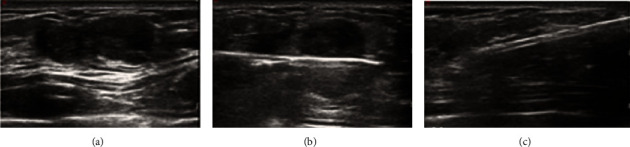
A lesion in a 36 years woman, which was demonstrated to be PTs by pathology. (a) Ultrasound imaging showed a hypoechoic mass; (b) the probe in the mass; (c) complete resection of the mass.
